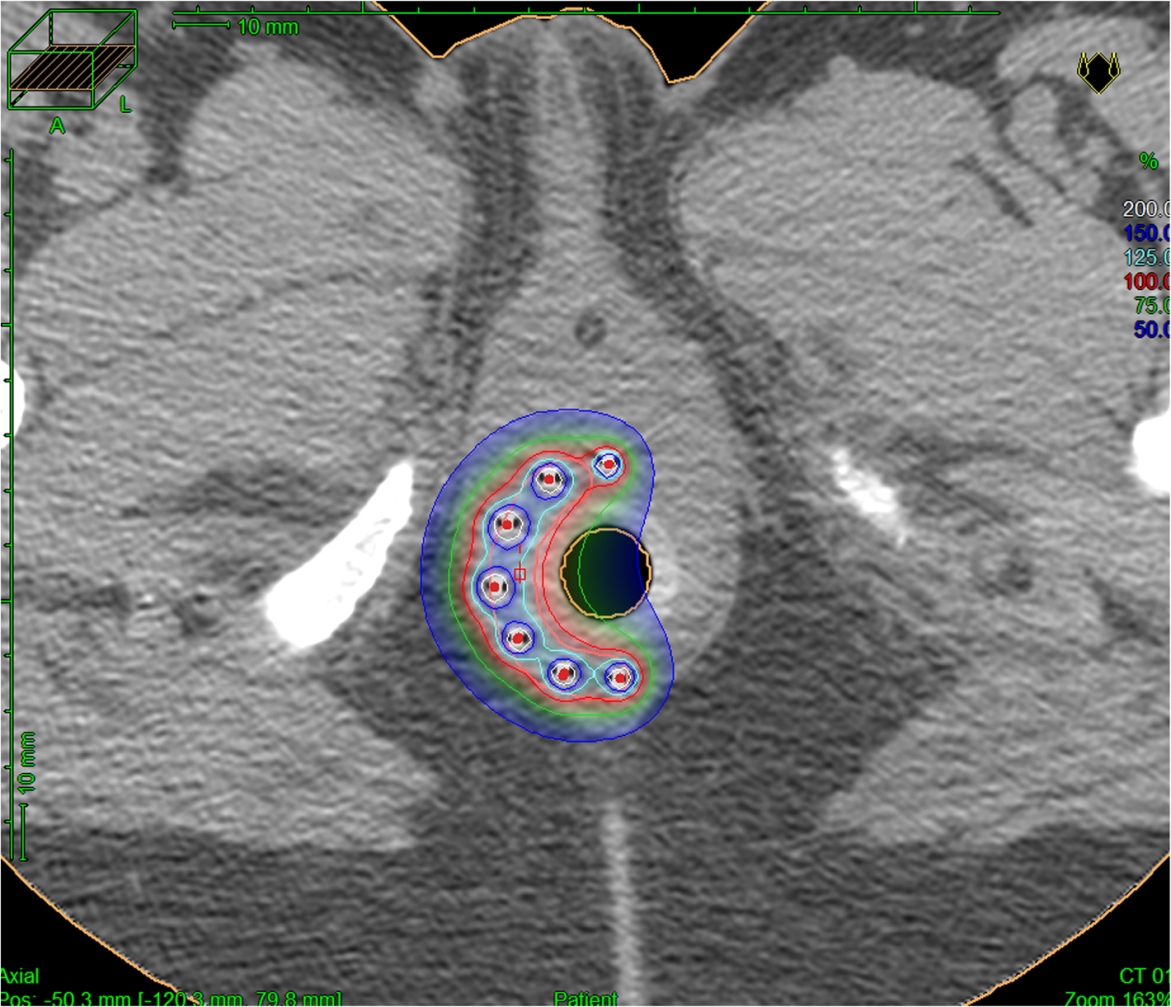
Dose distribution analysis on the post-implant CT-scan.
